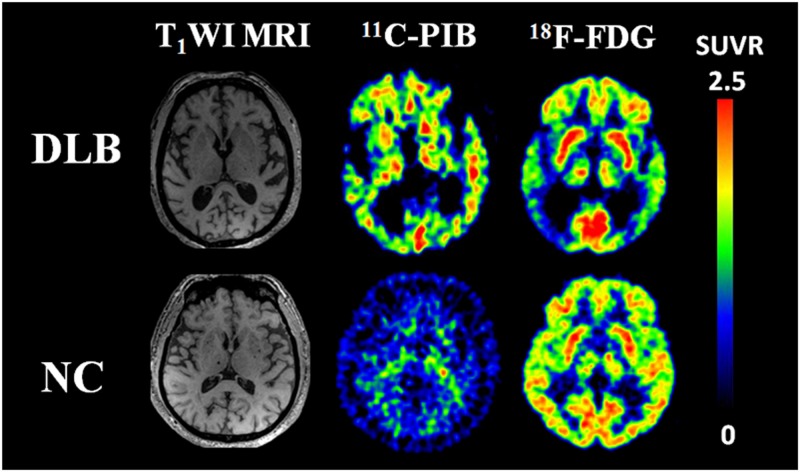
MRI (left), PiB (middle) and FDG (right) images of a Chinese DLB patient and a healthy control. PiB and FDG images are quantified by SUVR with the displayed color scales. In DLB patient, the clearance rate of radioactivity was slower symmetrically or asymmetrically in the cortex of the frontal lobe, parietal lobe, lateral temporal lobe, precuneus, posterior cingulate and occipital lobe.
